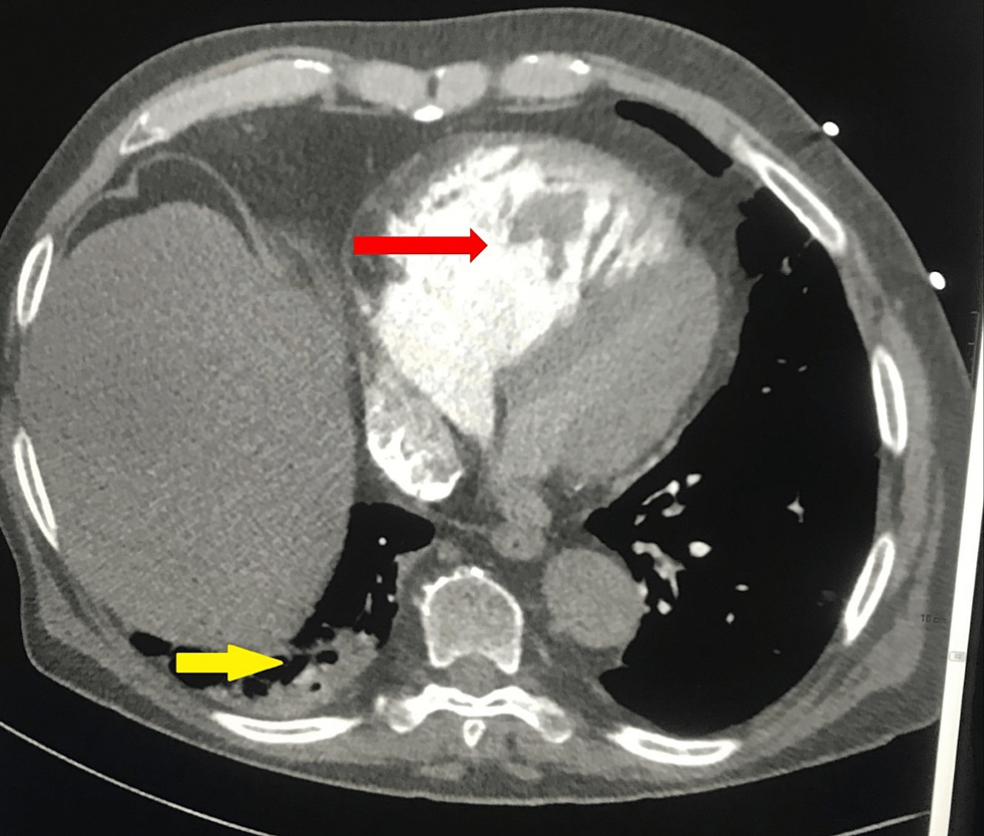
CT showing a red arrow pointing at the thrombus in the right ventricle and a yellow arrow pointing at consolidation in the right inferior lobe
